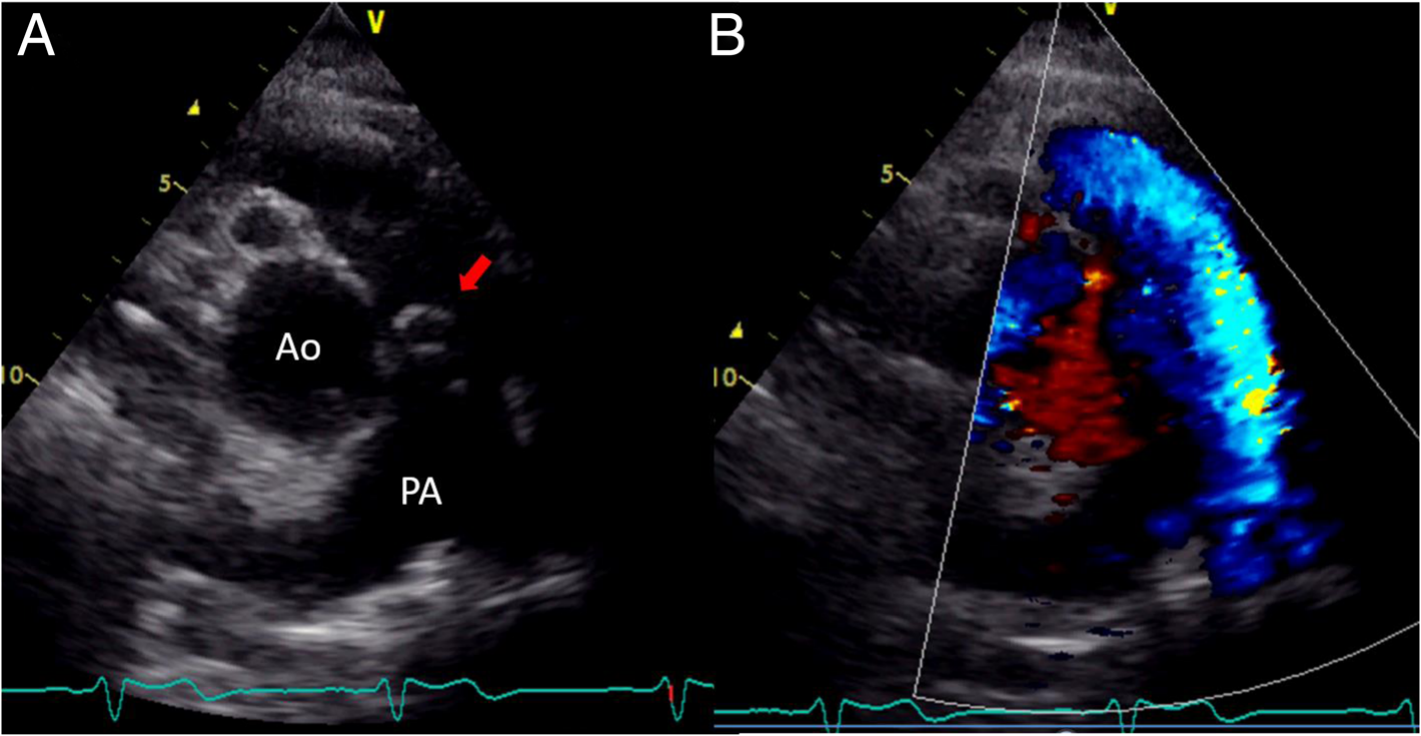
Post-operative echocardiogram. a Post-operative echocardiogram revealed the patency of the vascular prosthesis. b No stenotic blood flow was revealed in the color Doppler. Ao: aorta, PA; pulmonary artery, allow; vascular prosthesis
